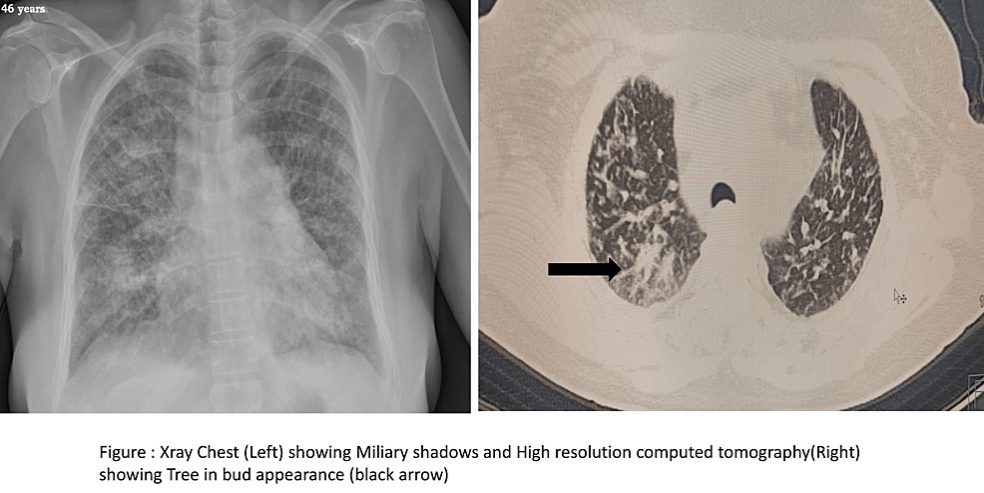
Chest X-ray and thorax HRCT showing radiological features of pulmonary tuberculosis. Tree-in-bud appearance (black arrow). HRCT: high-resolution computed tomography.
